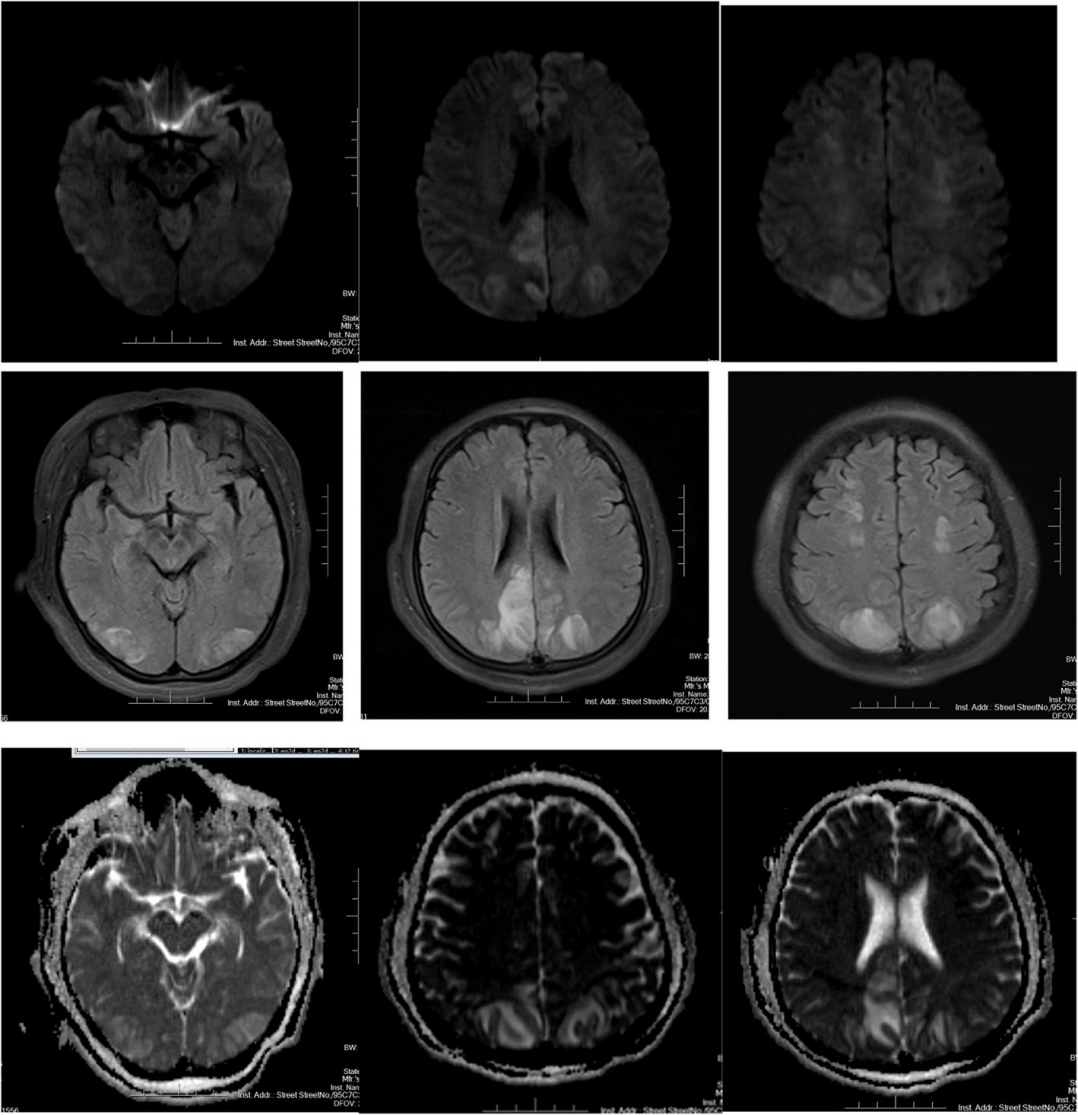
Brain MRI in a patient with Eclampsia with Hypothyroidism Complicated with Posterior Reversible Encephalopathy Syndrome (PRES). Legend: diffusion restriction on the diffusion-weighted sequences and apparent dispersion coefficient (Both sides)
